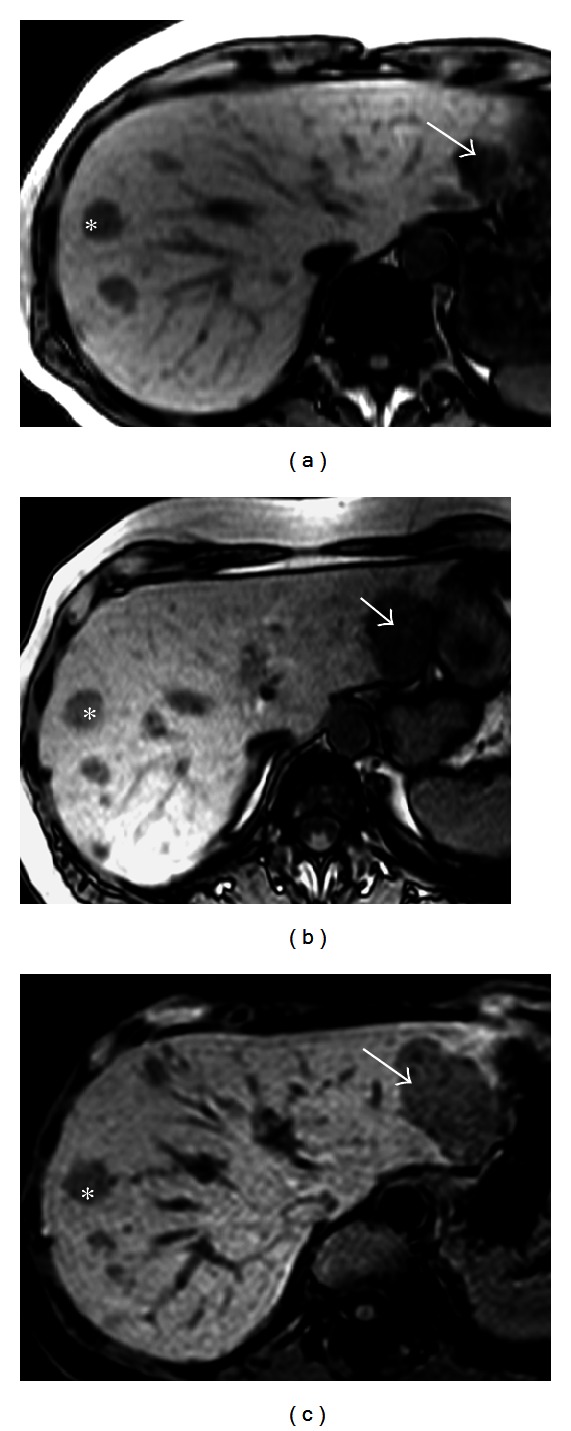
H-HCA size increment. Woman born in 1952. Abnormal liver function tests. Oral contraceptives 13 years, BMI 25.4 MRI adenomatosis, largest nodule 6 cm. Segmentectomies IV and VI 2001. Follow-up: increase size of one nodule Segmentectomy III, 2010. (a–c) Phased-opposed T1 weighted MR images in 2001 (a), 2007 (b), and 2010 (c) showing multiple hepatocellular adenomas showing hypointense because of the massive fat component. The nodule located in segment II (arrow) gradually increases in diameter between 2001 and 2010. As the 13 mm nodule located in segment VIII (asterisk), other nodules did not change their size.
